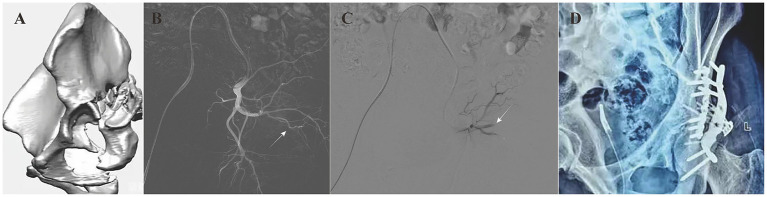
Representative case demonstrating anatomy-guided prophylactic superselective embolization in a complex acetabular fracture. (A) Three-dimensional CT reconstruction showing the acetabular fracture morphology and its anatomical relationship with the planned surgical approach. (B) Preoperative angiography demonstrating occult injury of a superior gluteal artery branch (white arrow). (C) Superselective catheterization and embolization of the injured superior gluteal artery branch, with the white arrow indicating the target vessel. (D) Postoperative radiograph following open reduction and internal fixation.
